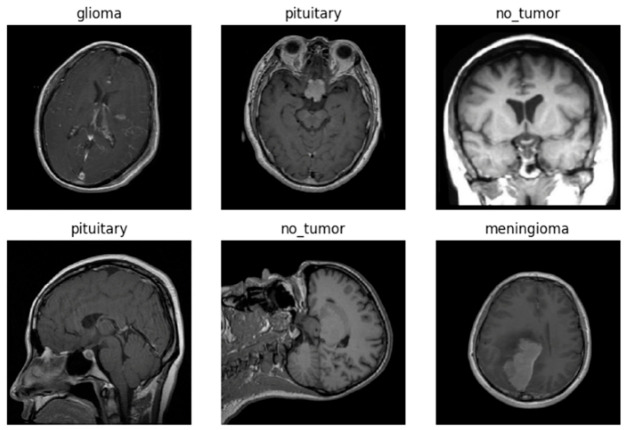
Sample visualization of brain MRI training data.
